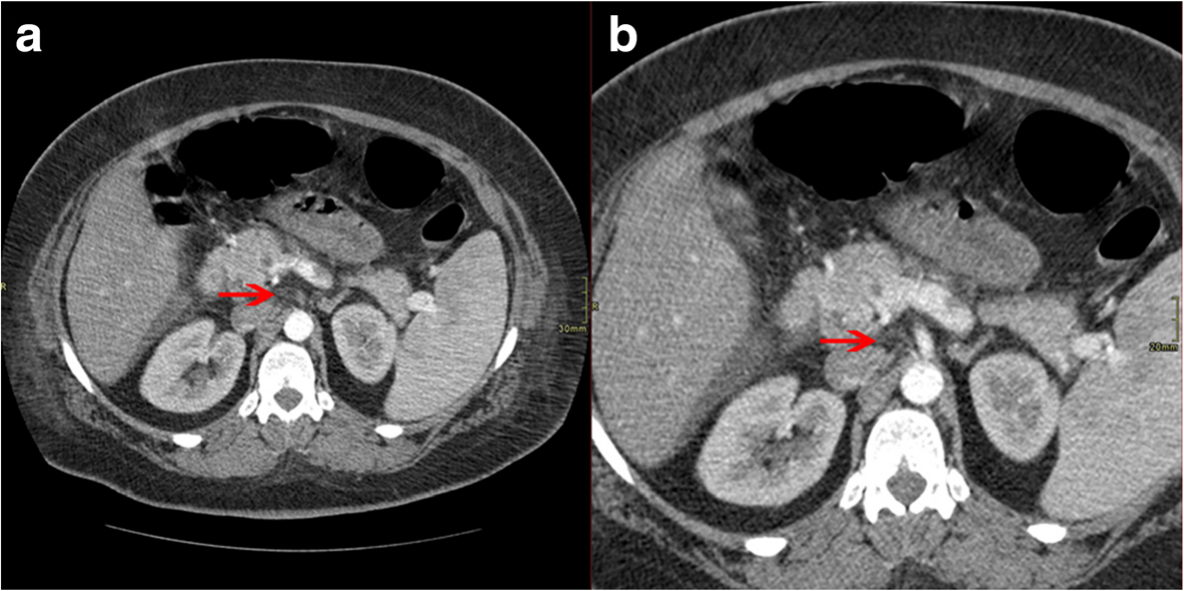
Abdominopelvic computed tomography scan. a, b Filling defect – thrombus is revealed in junction of liver–spleen axis and upper mesenteric vein (*red arrows*)
